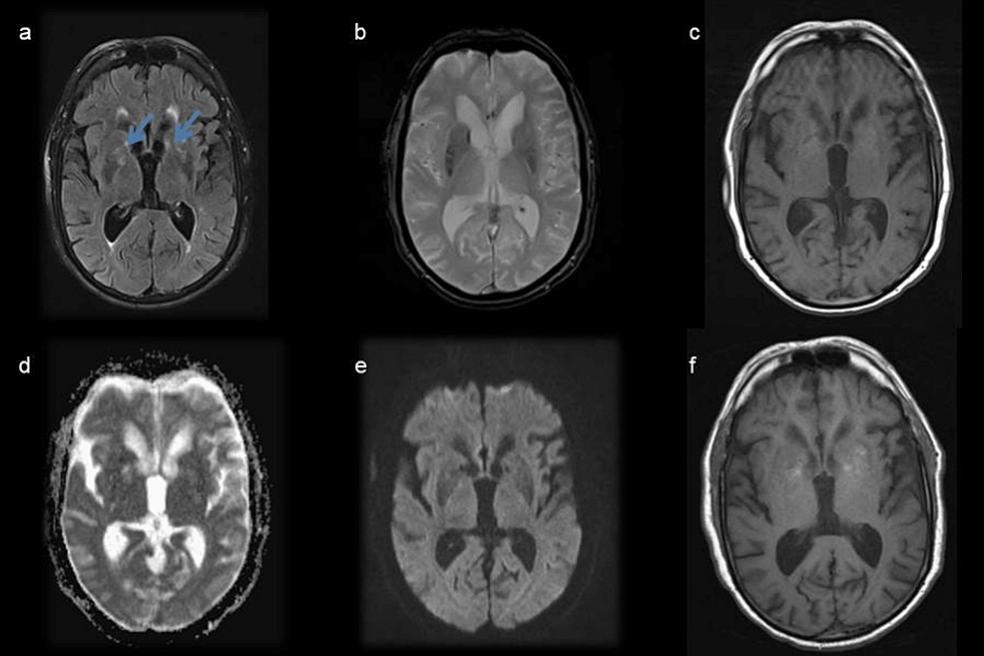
Brain magnetic resonance imaging at six weeks after initial symptoms showing attenuation of bilateral symmetrical heterogeneous lenticulocapsular and caudate nuclei hyperintensity (blue arrow) on T2/fluid-attenuated inversion recovery (a), without hyperintensity foci on T1 sequence (c), microhemorrhages (b), restriction to diffusion (d, e), or contrast enhancement (f).
